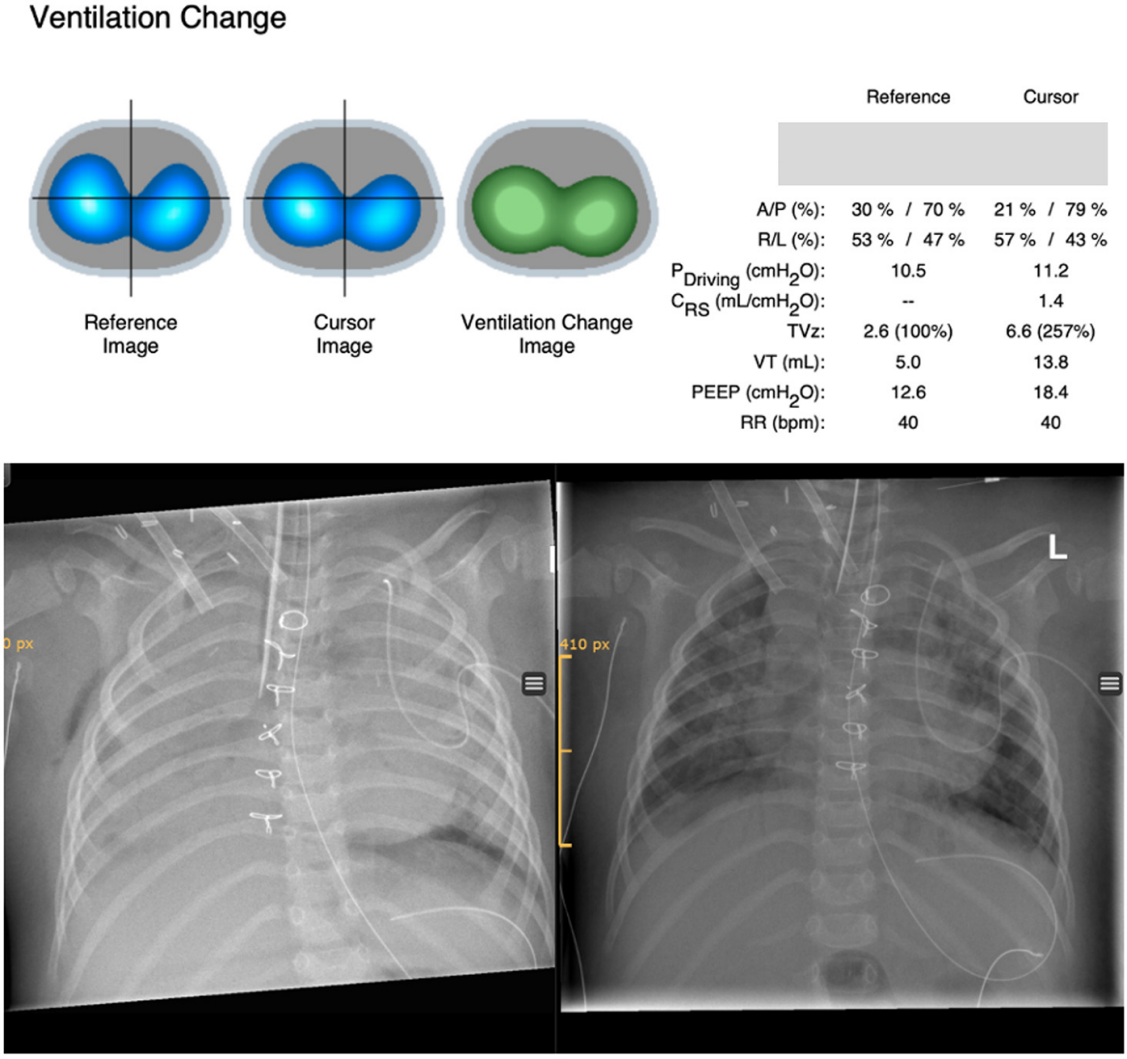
Ventilation changes derived from EIT are shown above. The reference image shows ventilation distribution before recruitment and the cursor image ventilation distribution after recruitment. The ventilation change image compares ventilation distribution before and after recruitment (reference image vs. cursor image) based on EIT pixels and displays changes in colors (green, gain in ventilation; red, loss in ventilation). Ventilator settings for paired time points and associated changes of different ventilatory and EIT parameters are shown on the right side comparing the same reference and cursor time points. Ventilation change after recruitment on ECMO with incremental/decremental PEEP titration up to 24 cmH_2_O showed a significant gain of ventilation (green) and improvement in tidal volumes. Chest x-ray before recruitment maneuver (left picture) and after recruitment (right picture). A/P, anterior/posterior; R/L, right/left; Pdriving, driving pressure; Crs, compliance respiratory system; TVz, tidal volume based on EIT signals; VT, tidal volume in milliliters.
